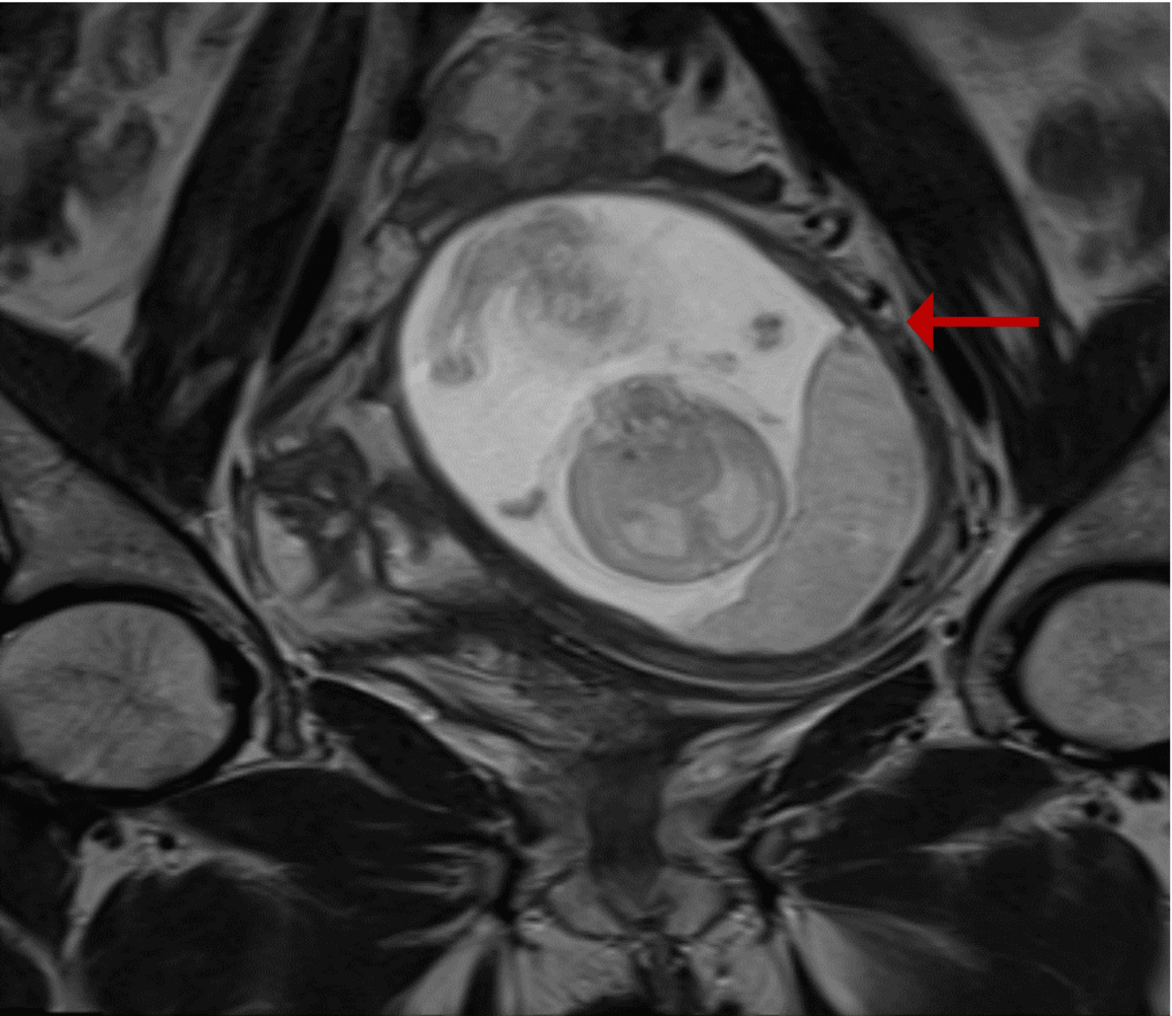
Focused coronal section of MRI pelvis showing the rudimentary horn with fetus and placenta (red arrow).
